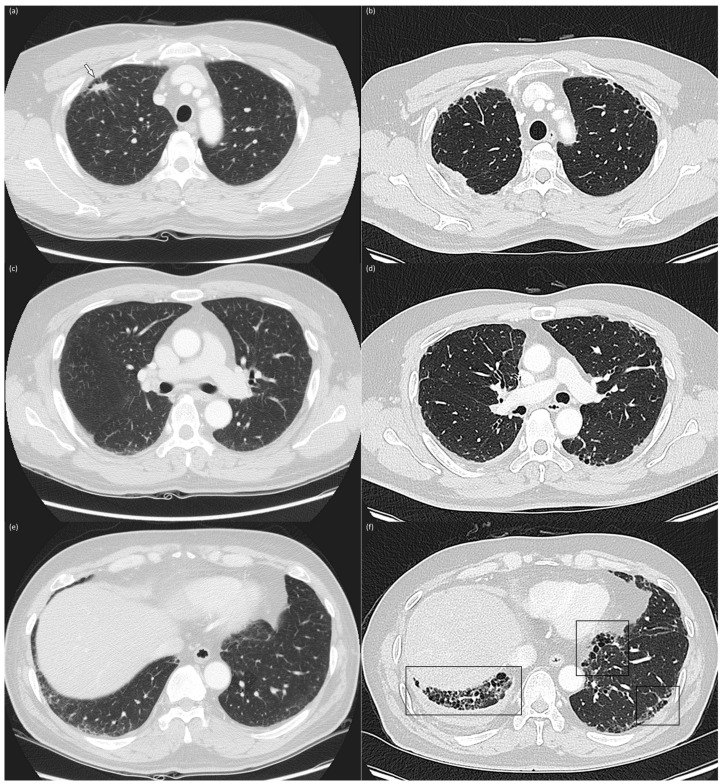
Baseline and 4-year follow-up chest computed tomography images Baseline (a,c,e) and 4-year follow-up (b,d,f) chest CT images in the upper (a,b), middle (c,d), and lower (e,f) lung zones of a 56-year-old, male current smoker with a 60 pack-year smoking history. The baseline CT reveals subpleural reticulation and mild traction bronchiolectasis in both lungs, suggesting the presence of fibrotic ILAs. A 1.5 cm-sized nodule (arrow) was identified in the right upper lobe and confirmed as adenocarcinoma (a). The patient underwent right upper lobe lobectomy and adjuvant chemotherapy with cisplatin and paclitaxel. The 4-year follow-up CT reveals increased subpleural reticulation, traction bronchiectasis, and honeycombing (box), predominantly in lower lung zones, indicating ILA progression.
